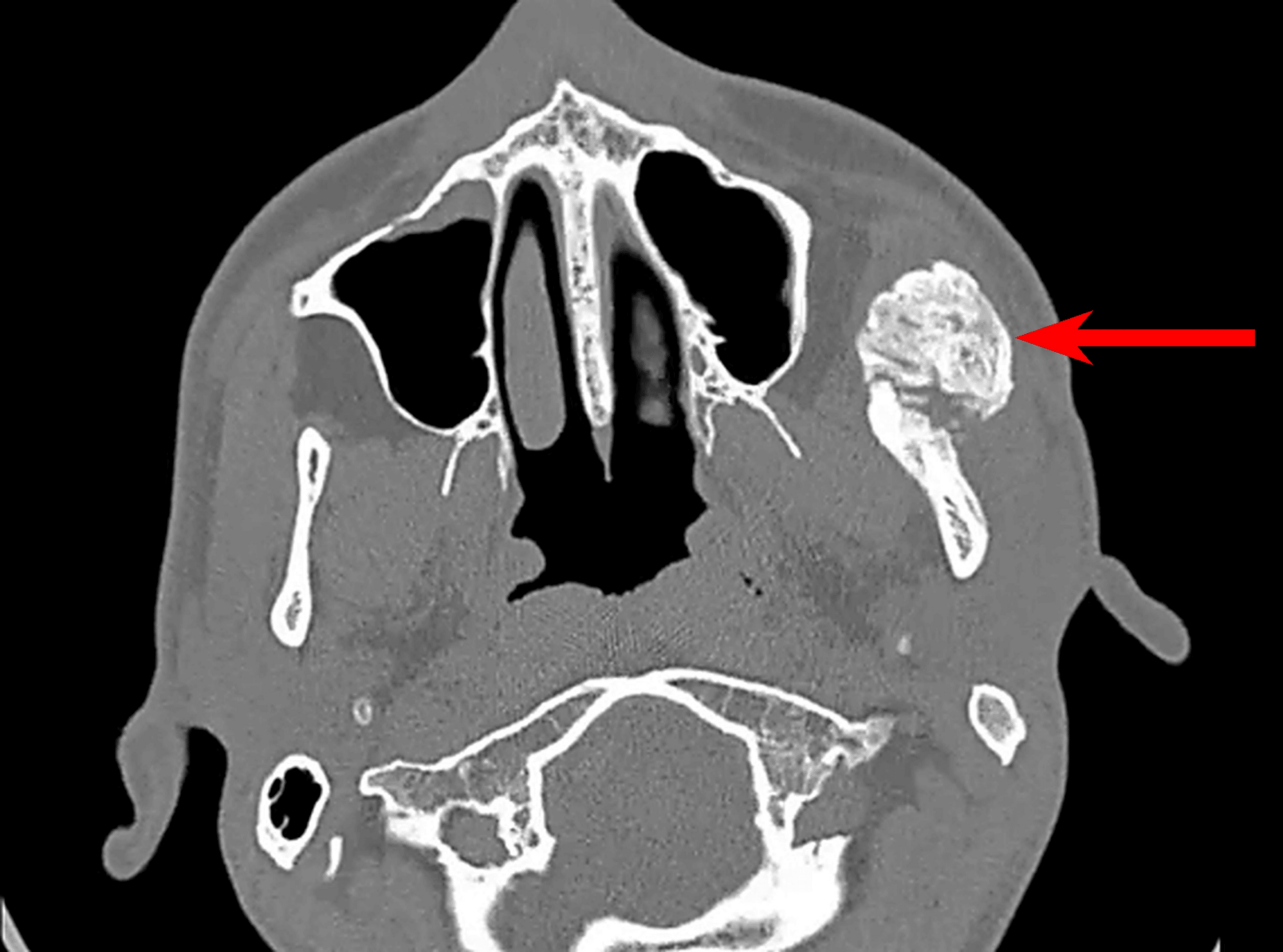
Axial CT (bone window) demonstrating heterotopic ossification of the left masseter (red arrow).
